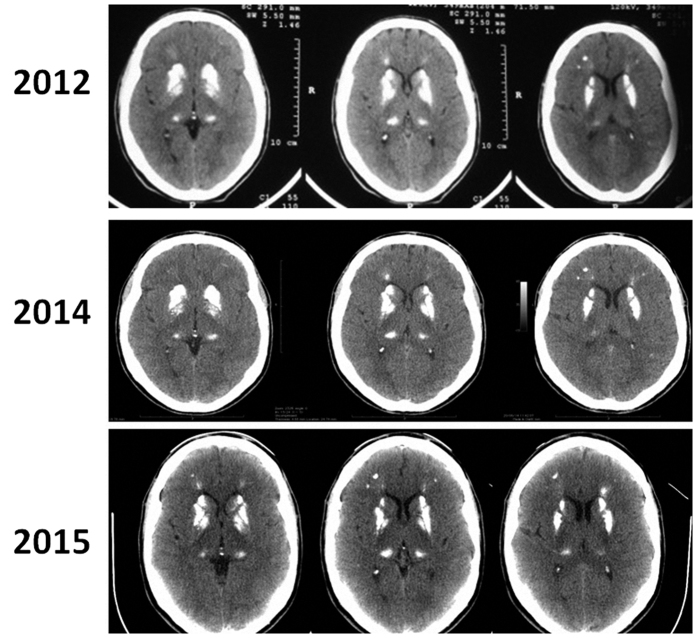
Details case 7. It is possible to see three CT separated by three years (2012, 2014 and 2015). No specific image change can be recognized, although symptoms improved after alendronate use.
